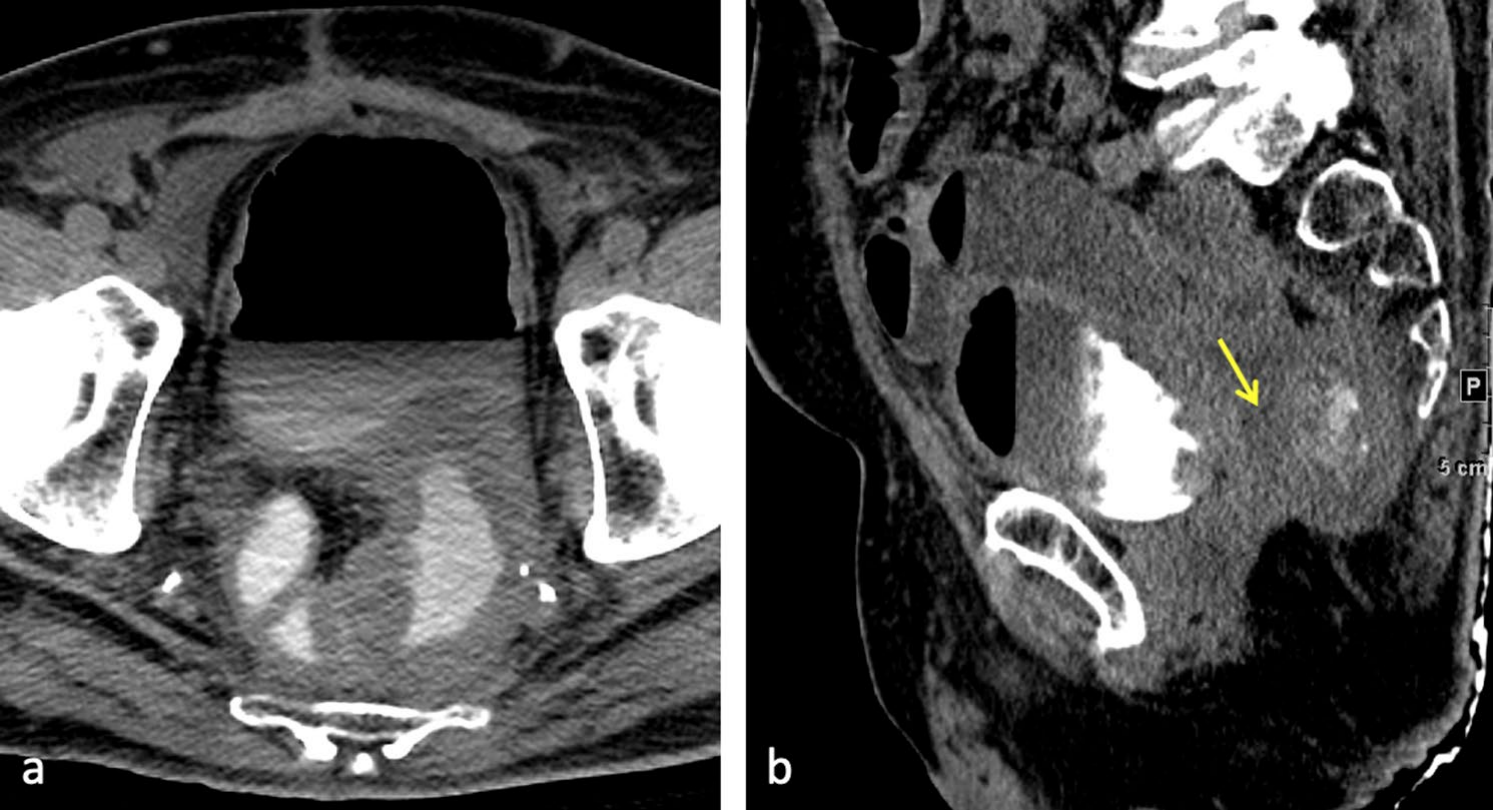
82-year-old male with history of prostate cancer and rectal cancer status post abdominoperineal resection. Axial a and sagittal b contrast-enhanced CT cystogram demonstrates contrast in the rectum with a fistulous connection to the air-filled bladder (arrow), compatible with a rectovesical fistula
